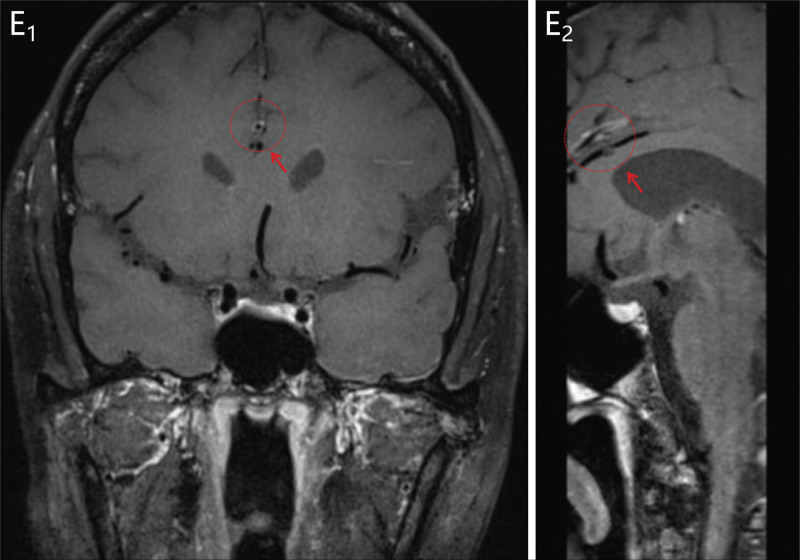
High-resolution Magnetic Resonance Image (HR-MRI). (E_1_ and E_2_) The high-resolution magnetic resonance image (HR-MRI) shows vascular inflammatory changes in the anterior cerebral artery with ring enhancement, suggesting arterial vasculitis. There were no atherosclerotic changes observed in the right posterior cerebral artery.
